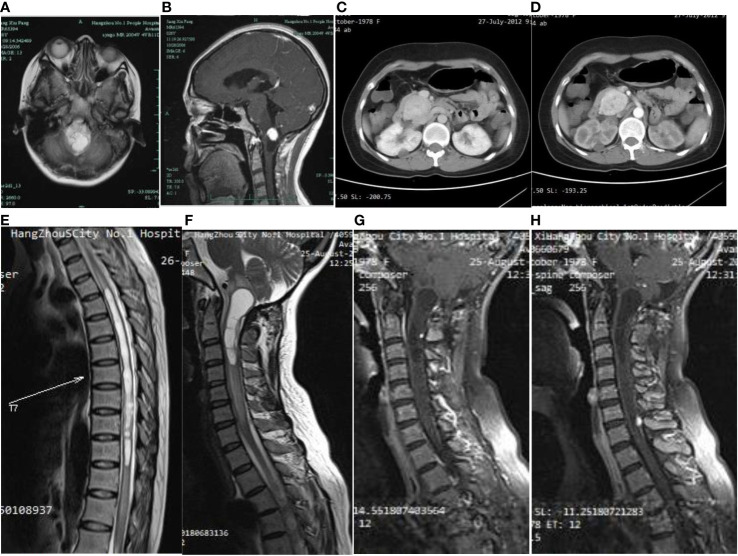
On first admission to the hospital, the brain MRI cystic-solid occupying lesion in the medulla oblongata (A), the solid part showed significant enhancement, while the cystic part was not enhanced (B). Abdominal CT showed an elliptical soft tissue density shadow over the head of the pancreas (C), approximately 4.6×3.6 cm in size, with significant enhancement and several small cyst-like non-enhancing hypodensities in the pancreas (D). A long T2 edema band is seen around the thoracic medulla, which ranges from T4 to T12 segments. Long T1 (E) and long T2 (F) signal in the medulla oblongata and upper cervical medulla stripes, and foci of punctiform seen after enhancement (G). A round dotted lesion of the posterior spinal cord of the seventh cervical medulla with visible enhancement and localized spinal tail sign (H).
